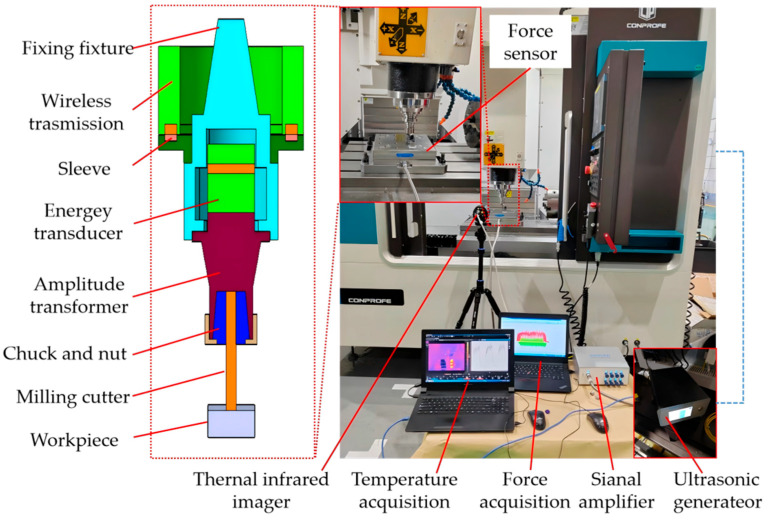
Ultrasonic vibration milling test setup.
